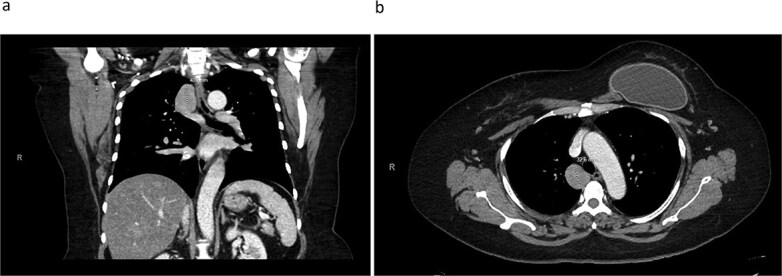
CT thorax revealing a 4.1 × 3.3 cm azygos vein aneurysm. a) Coronal view. b) Transverse view.
